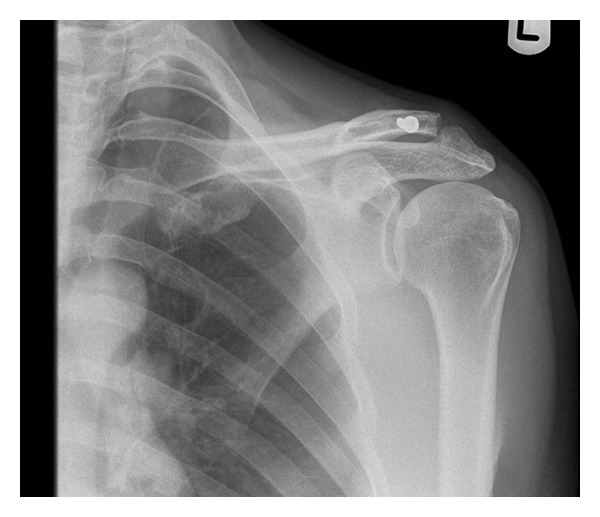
Postoperative AP radiograph showing the reduced joint.
